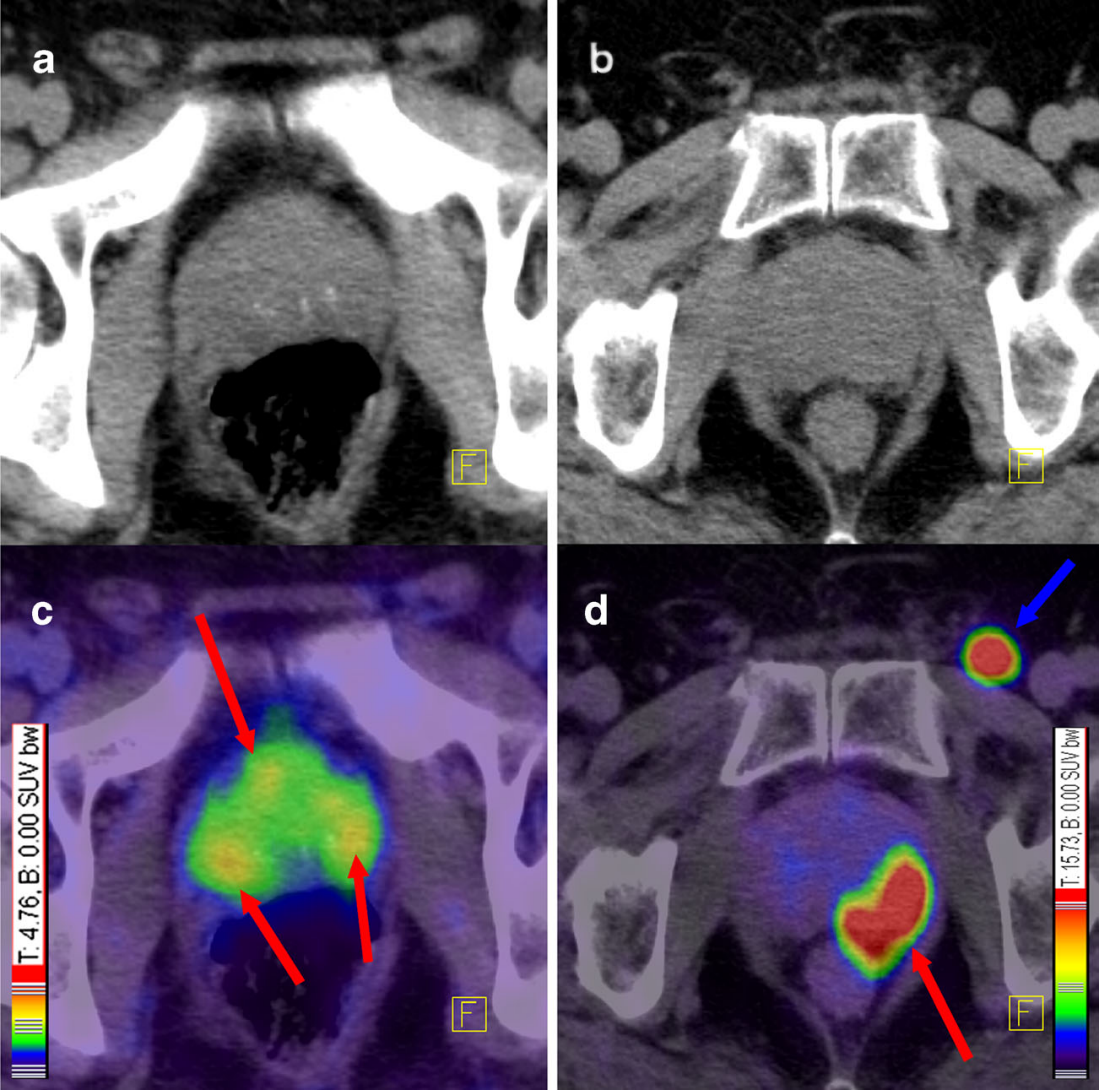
A patient with multifocal PCa (a, c) and another patient (b, d) with unifocal PCa with a rarely seen inguinal lymph node metastasis. *Red arrows* point to PCa within the prostate gland and *blue arrow* points to an inguinal lymph node metastasis. Both patients had GSC 7, although the tumours present with different contrast. Colour scales were automatically produced by the PET/CT machine. a Low-dose CT of the patient with a multifocal PCa, c corresponding fusion of PET and low-dose CT 1 h p.i., b low-dose CT of the patients with the unifocal PCa, d corresponding fusion of PET and low-dose CT 1 h p.i.
